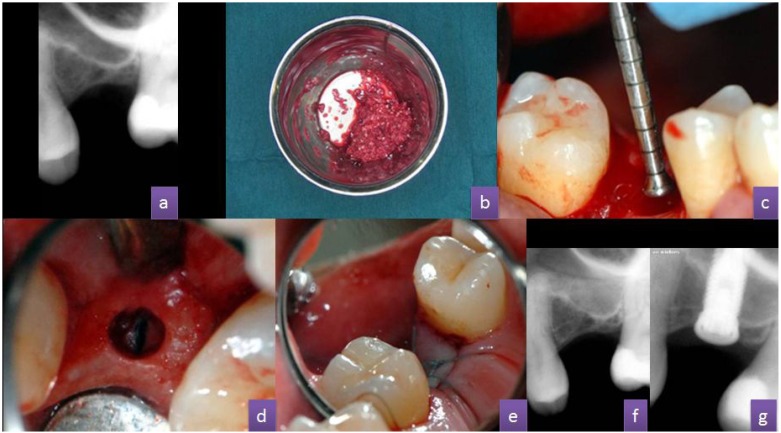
(A) Preoperative periapical X-ray, (b) Mixing blood collected from the edges of the incision with augmentation material (Biogen™, Bioteck, Italy) (c) TKW5 tip, also called 'Trumpet', (d) Hole created on the ridge for subsequent use of a TKW5 tip, (e) 4/0 silicone-coated polyester suture, (f) Periapical X-ray of maxillary sinus with augmentation material inserted, (g) Periapical X-ray of the maxillary sinus with augmentation material and implant in place.
